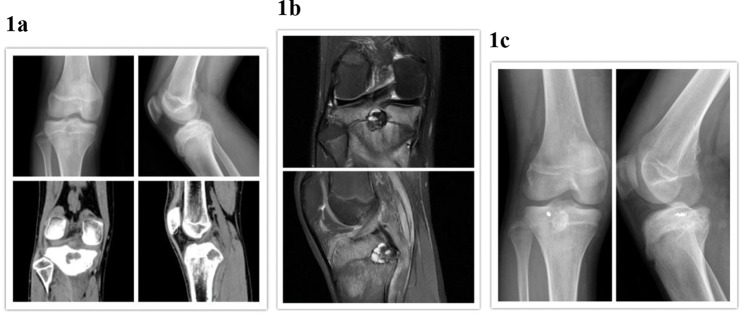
Male, 15, right knee pain for 3 months. 1a Preoperative X-ray films and CT scans showing a round osteolytic focus below the right tibial intercondylar posterior cruciate ligament just below the circular osteolytic lesion. There was sclerosis at the boundary, and calcification was observed in the lesion. 1b Preoperative MRI scans showing an inflammatory reaction around the lesion and edema of the surrounding bone marrow and soft tissues. 1c X-ray films at four months after the operation suggesting that the grafted bone granules in the lesion had integrated with each other, resulting in an unclear boundary between the granules and the host bone.
